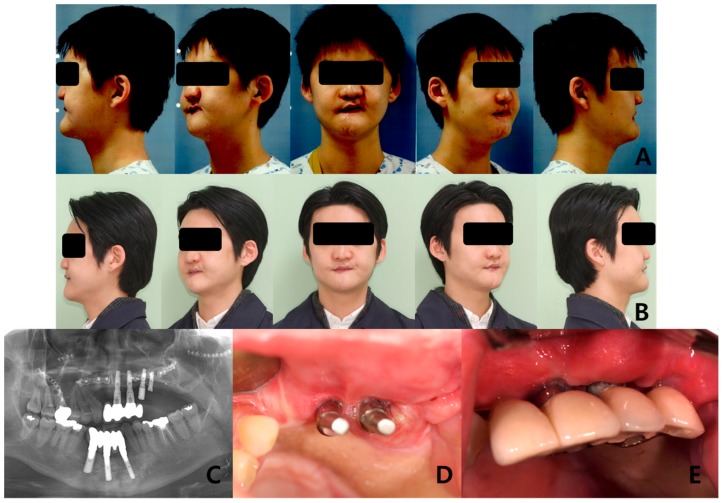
Long-term follow-up of the patients who received prosthetic rehabilitation with dental implant after the fibula free flap: (A) Preoperative clinical photos, (B) postoperative clinical photos after 4 years, (C) panoramic radiograph after implant treatment, (D) connection of the customized abutment, and (E) final prosthesis.
